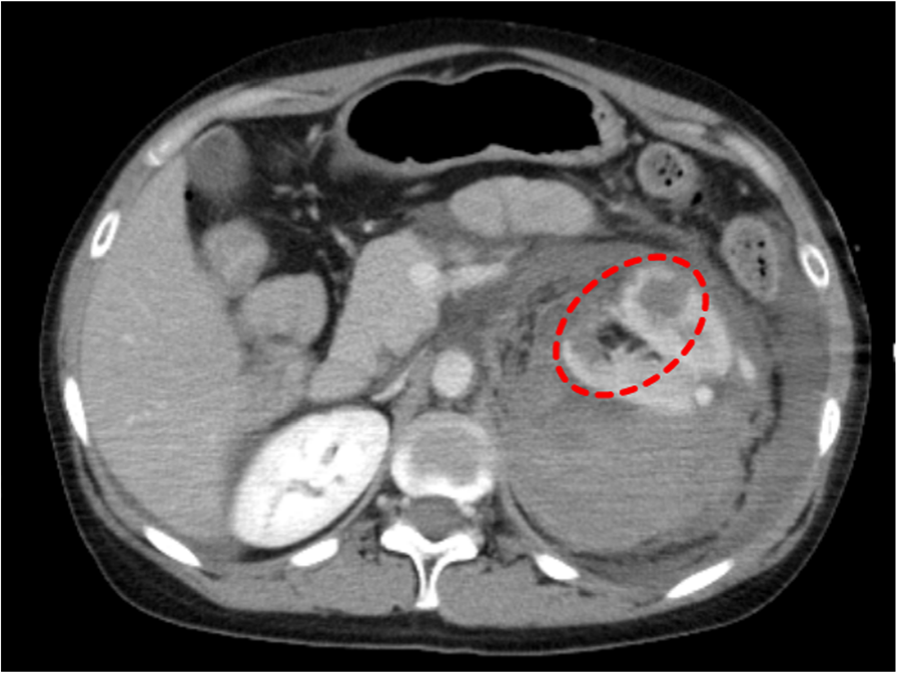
Contrast-enhanced CT. Contrast-enhanced CT shows two potential sites of intrarenal rupture of arteries (circle)
